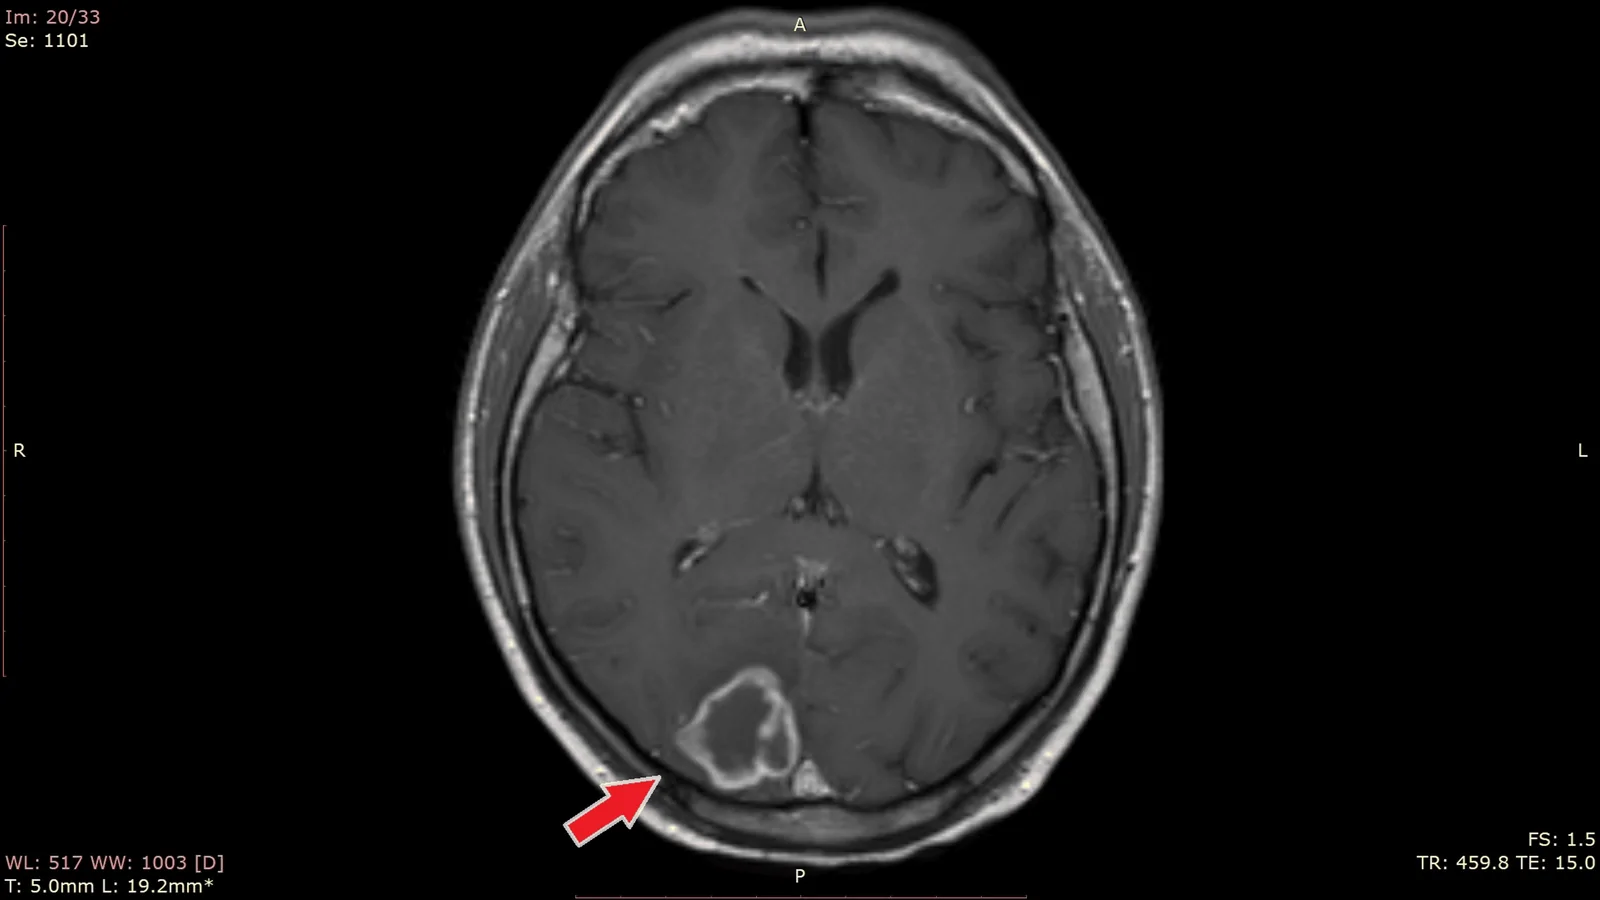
Initial 2024 MRI. T1-weighted contrast-enhanced MRI with gadolinium showing a metastatic lesion in the right occipitoparietal region (red arrow).
